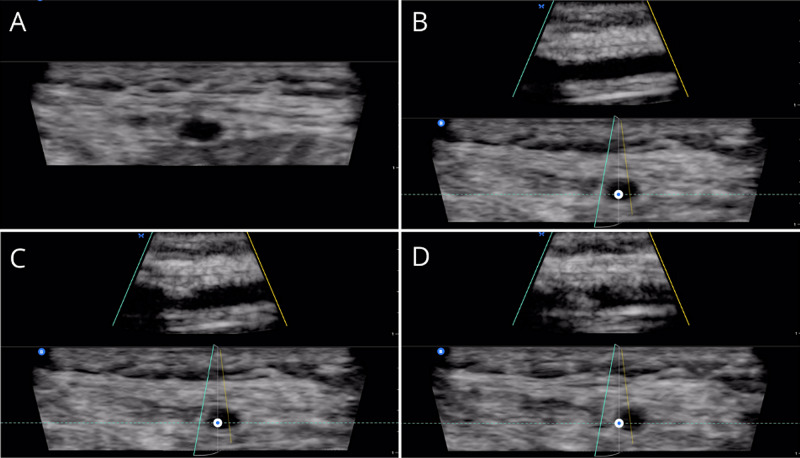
Views of the radial artery during sequential stages of arterial line access and insertion with the Butterfly IQ+ handheld ultrasound device A: The vessel in short-axis view. B: The vessel in biplane view, both long and short. C: The vessel in biplane view with needle entering the vessel. D: The vessel in biplane view with a needle within the vessel.
